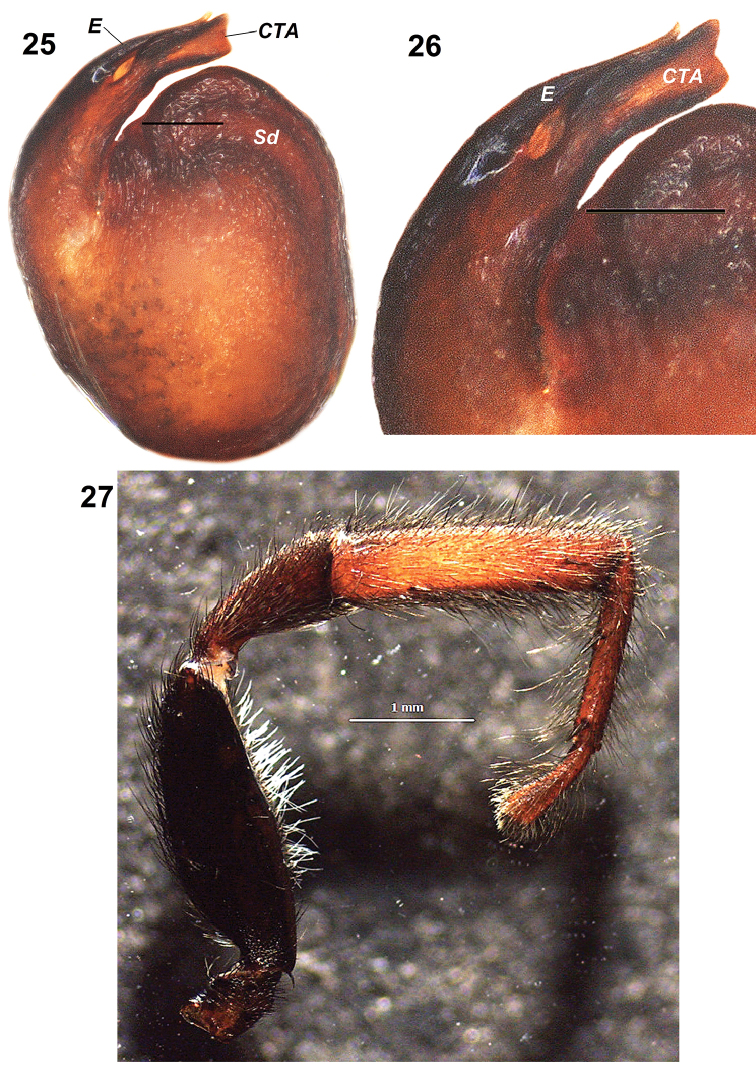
Pelleneshimalaya* sp. n., holotype male. 25 male left palp, ventral view 26 same, ventro-lateral view 27 left leg I, prolateral view. Scale bars: 0.1 mm (25–27). Abbreviations: CTA – compound terminal apophysis; E – embolus; RTA – retrolateral tibial apophysis; Sd – sperm duct.
